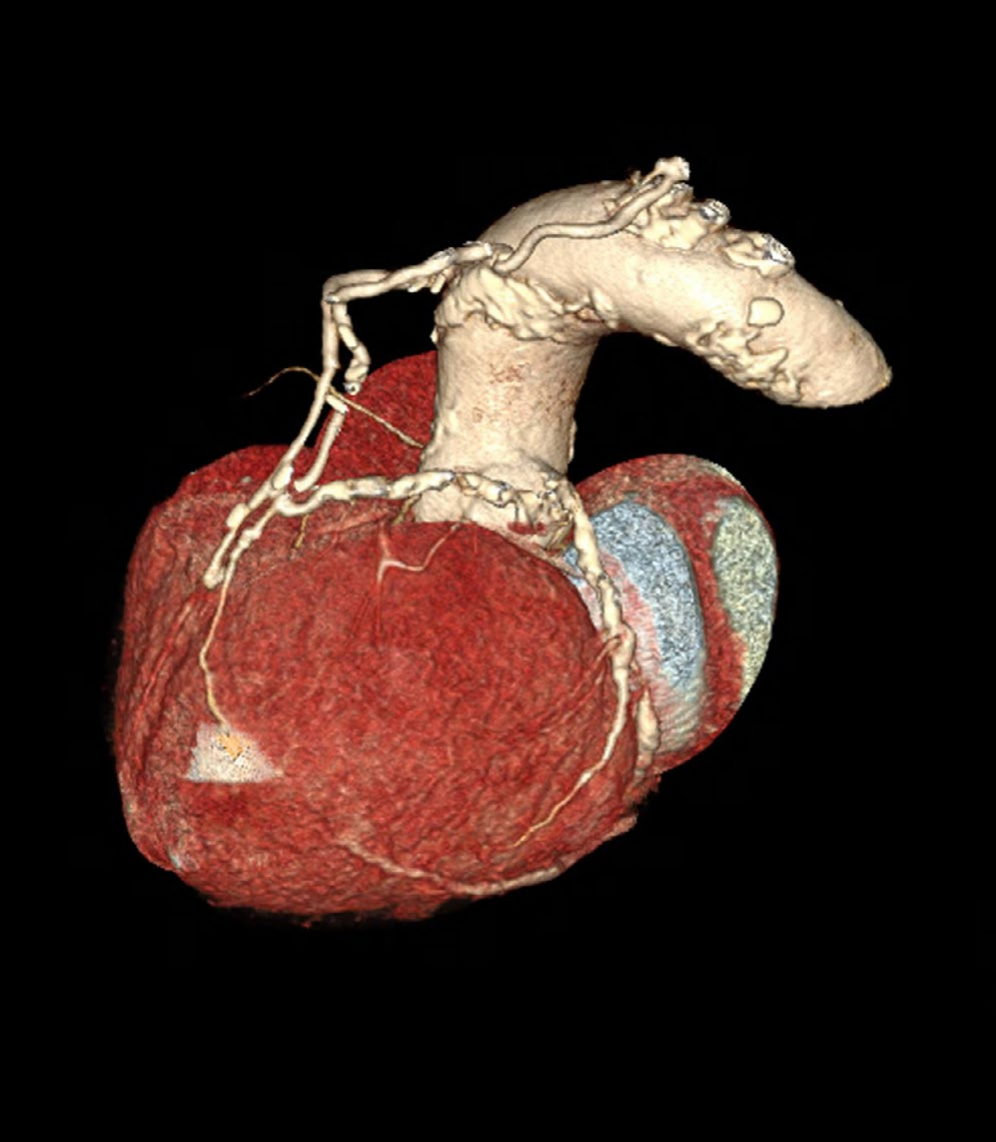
Coronary CTA, 3D reconstructions by dedicated software, confirming GCAA of RCA.
